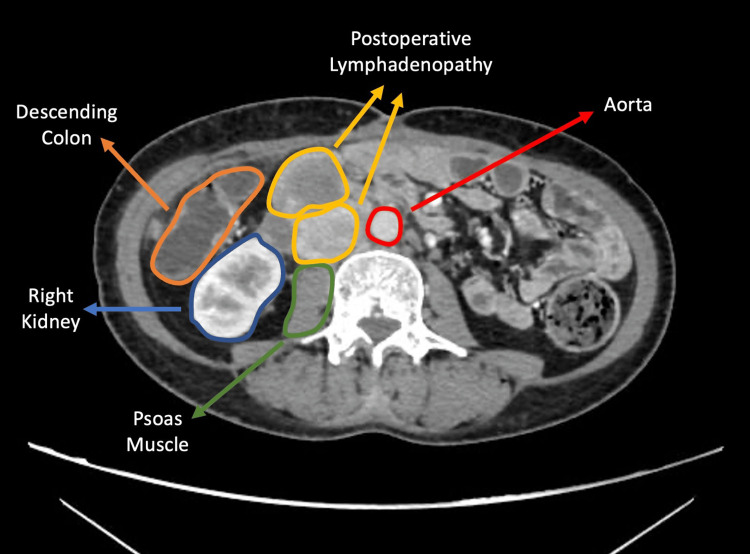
Contrast-enhanced axial CT image showing retroperitoneal lymphadenopathy Lymphadenopathies are seen anterior to the psoas muscle at the level of the renal hilum
